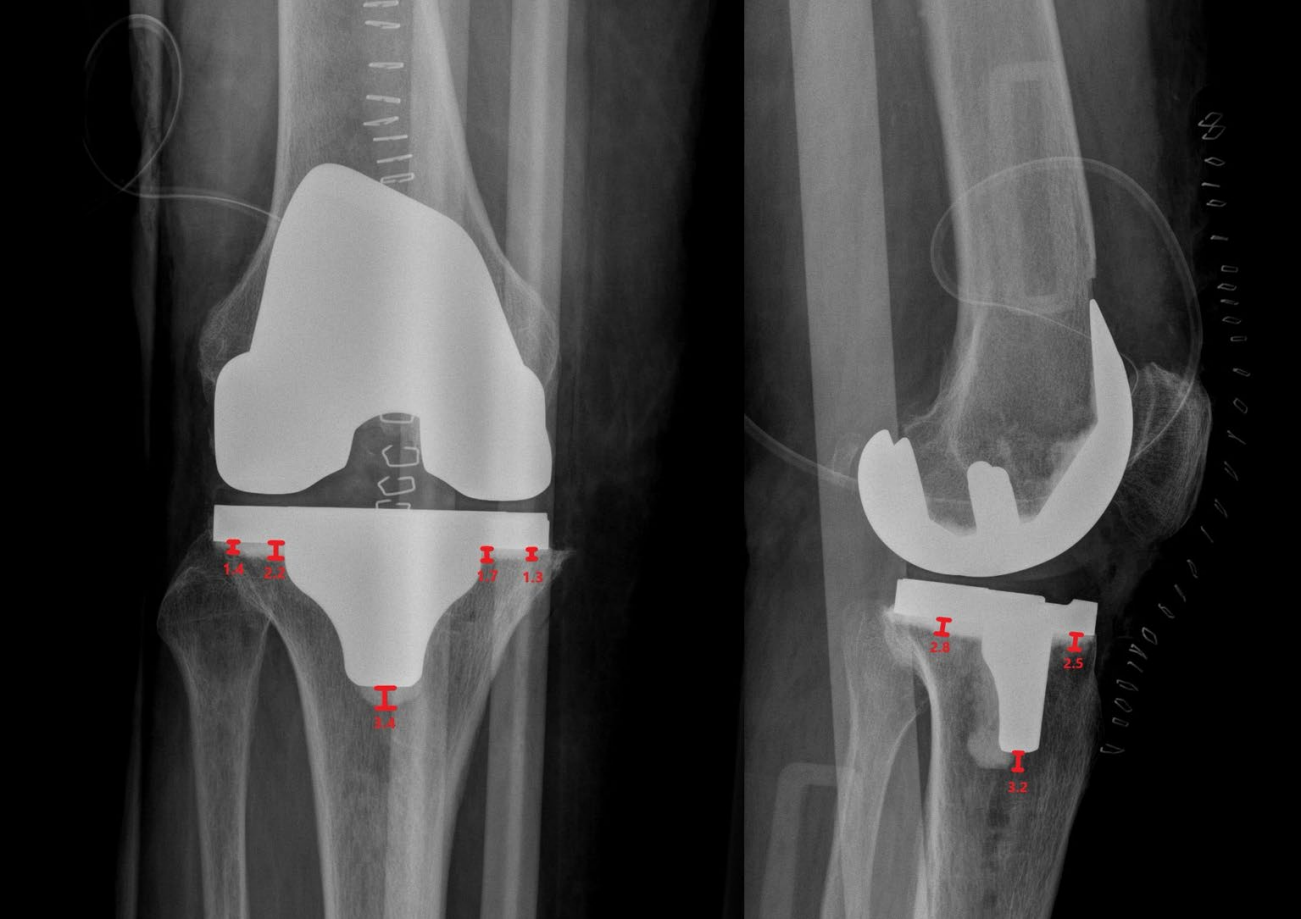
Radiographic measurement of cement penetration in both AP and lateral views in total knee arthroplasty
